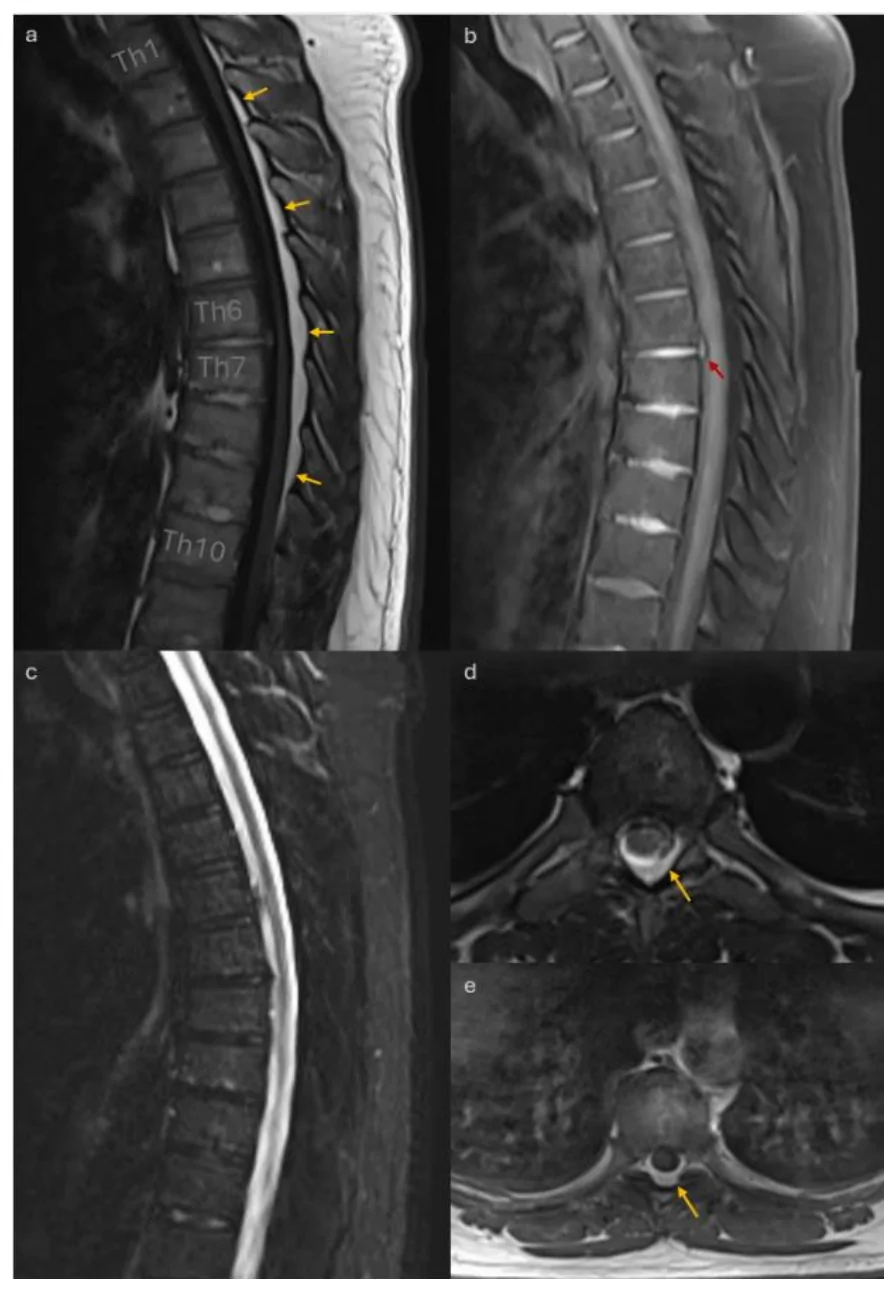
MRI of the thoracic region. Yellow arrows—epidural adipose tissue; Red arrow—Th6/Th7 intravertebral disc herniation. (a)—T1WI sequence, sagittal view; (b)—T1 Fat Saturation sequence, sagittal view; (c)—STIR sequence, sagittal view; (d)—T2WI sequence, transverse view, contrast enhancement; (e)—T2WI sequence, transverse view.
